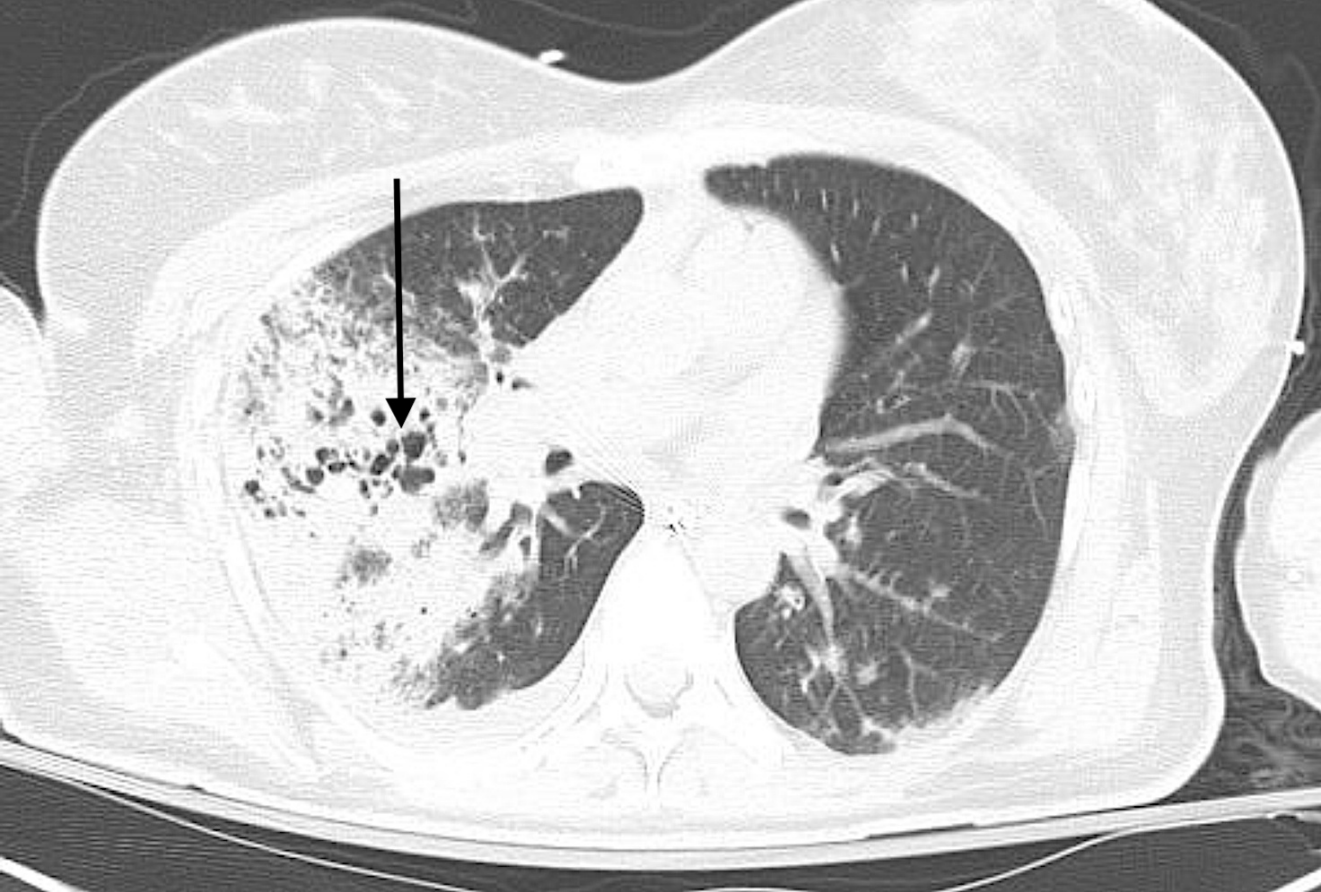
Computed tomography (CT) chest (axial view) showing cystic destruction of the lung parenchyma (arrow).
